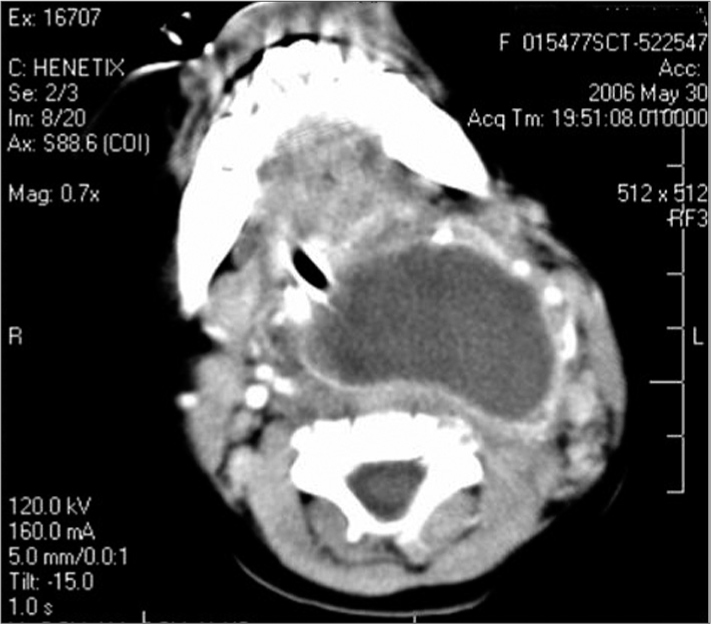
Large retropharyngeal abscess seen in computed tomography (CT).
